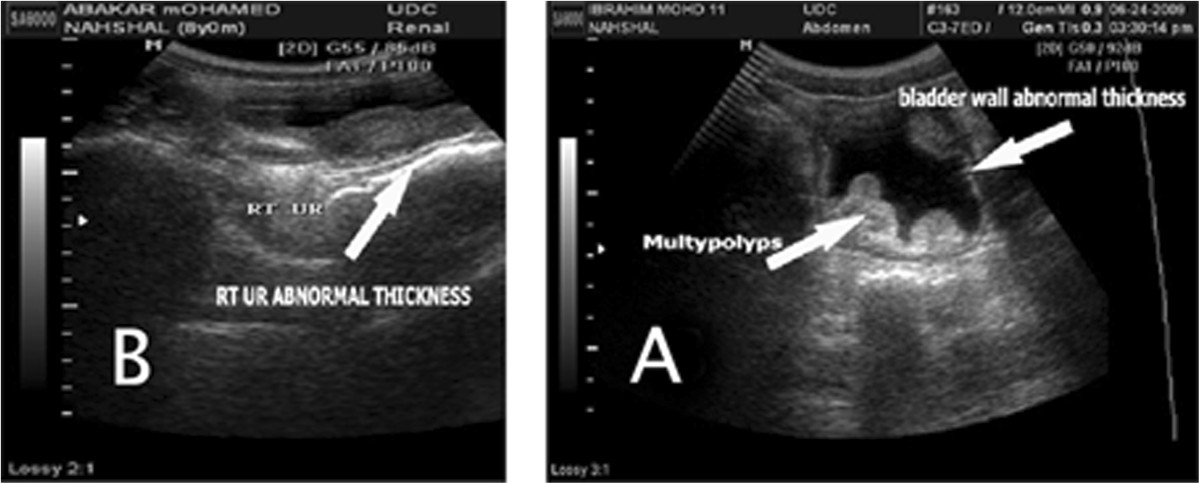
Shows Ultrasound Image. A: shows thickness on wall of bladder, polyps and calcification B: shows thickness of ureter 6 mm and Ultrasonographic examination was done by experienced radiologist.
